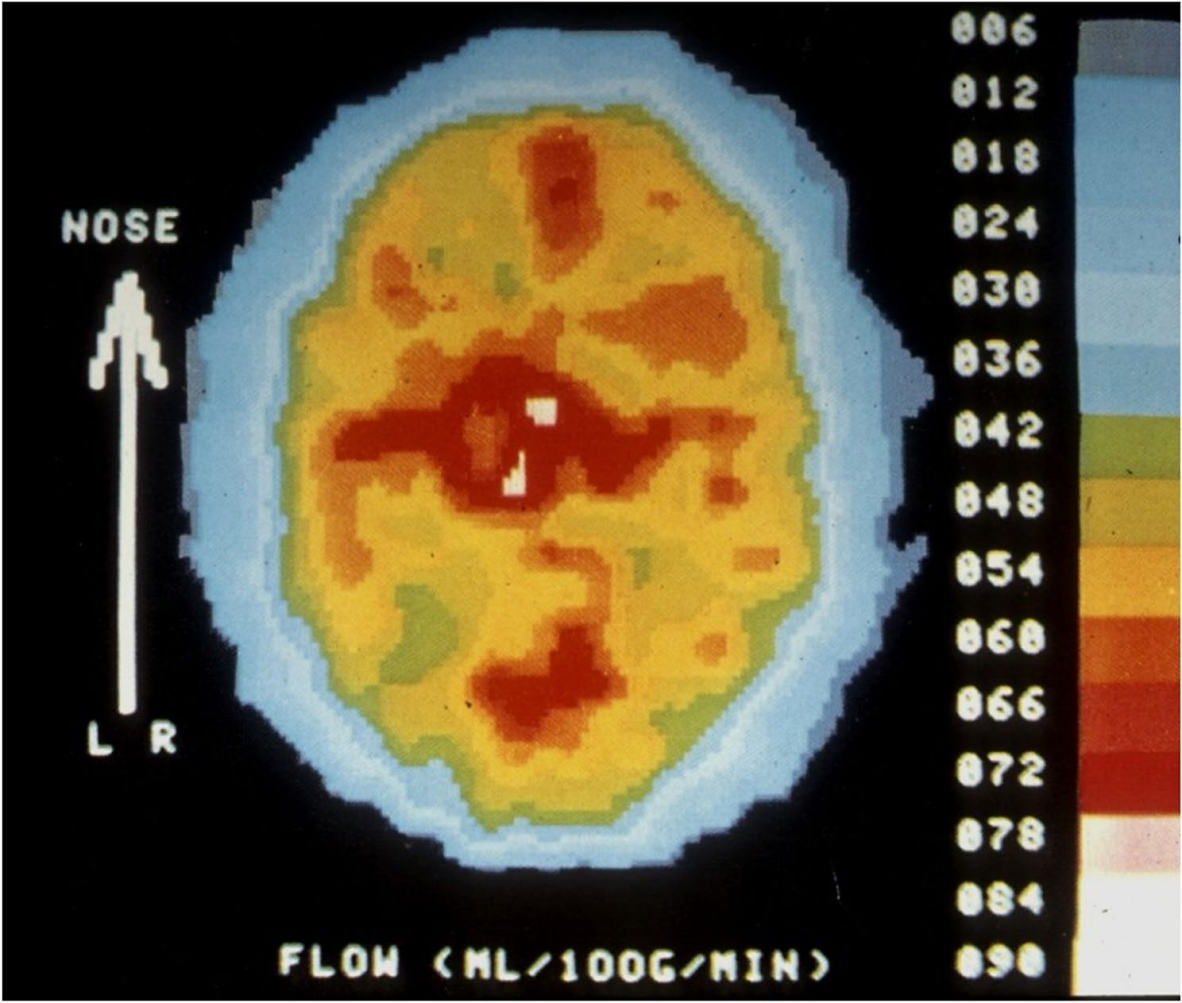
SPECT study of rCBF during an attack of MO. In all such cases rCBF remained normal and symmetrical with the resolution of the technique available that had been able to show focal changes in MA in every case. Subsequent studies with the higher resolution of PET has shown activation in the pain matrix secondary to the pain but nothing that resembles CSD. Modified from [5]
